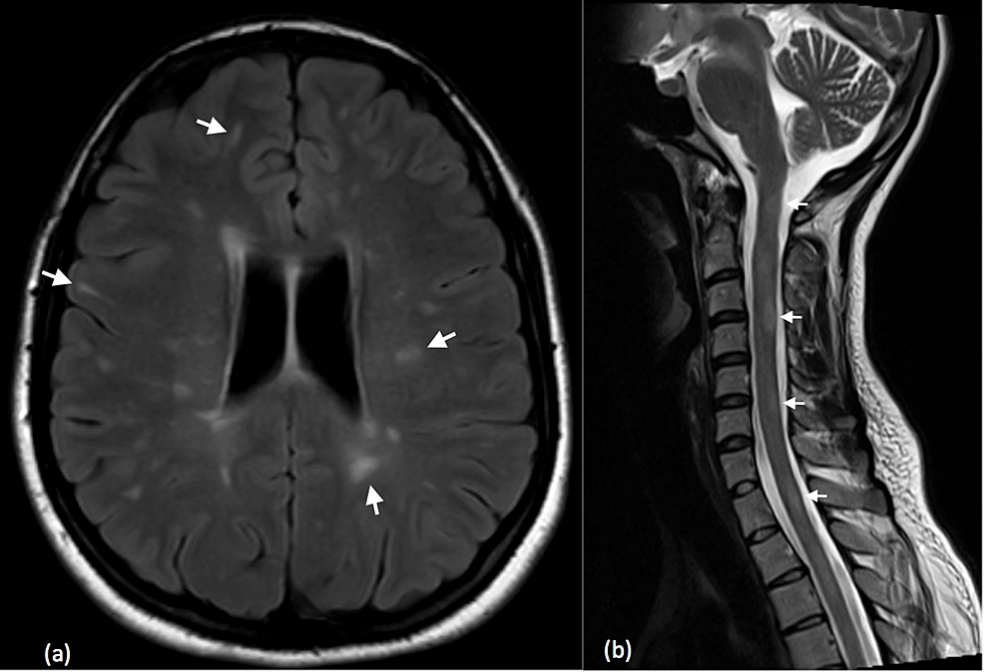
(a) Axial FLAIR image of the brain demonstrating multiple bilateral periventricular, deep white matter and subcortical hyperintense lesions (small white arrows), (b) sagittal T2-weighted images of cervical spine demonstrating multiple hyperintense intramedullary lesions (small white arrows) at different levels. FLAIR: fluid-attenuated inversion recovery.
